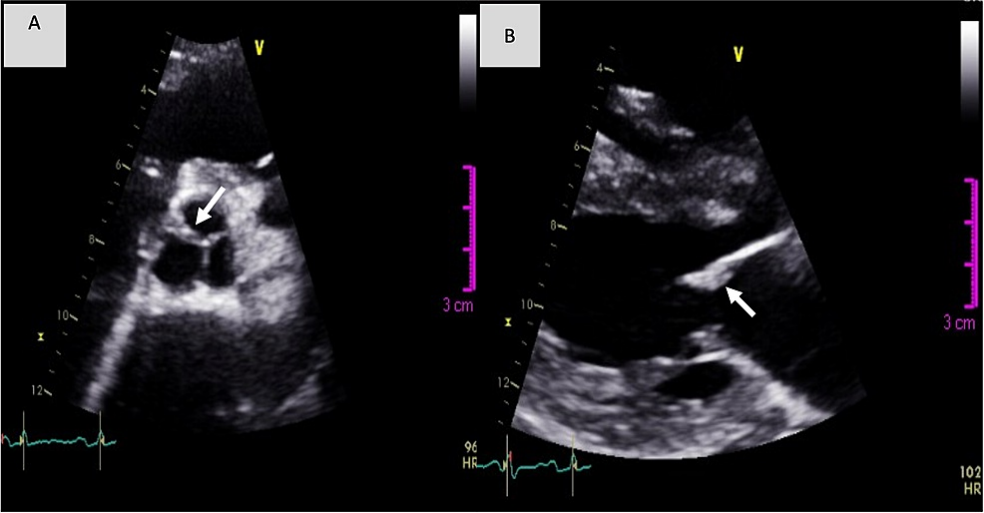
(A) Small vegetations on the aortic valve and (B) in the anterior mitral leaflet in transthoracic echocardiogram (arrows)
